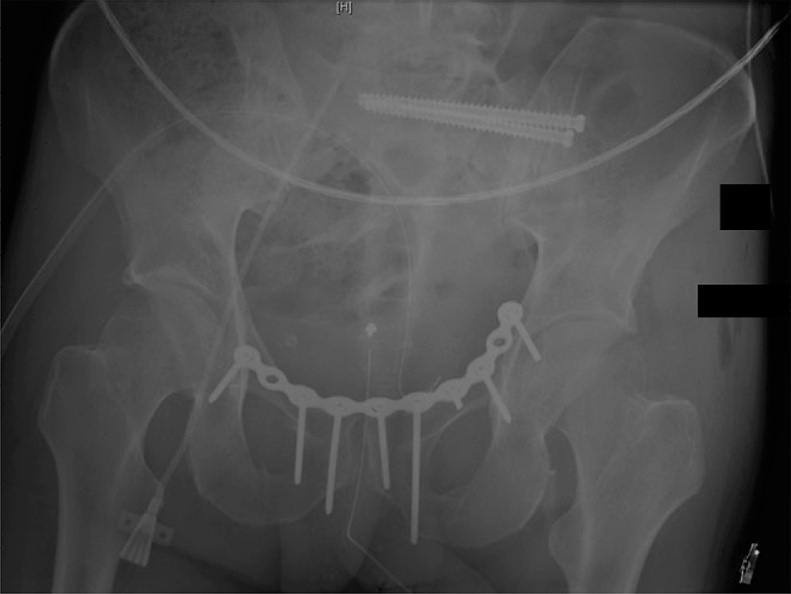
Pelvic anteroposterior X-rays taken after surgery.
